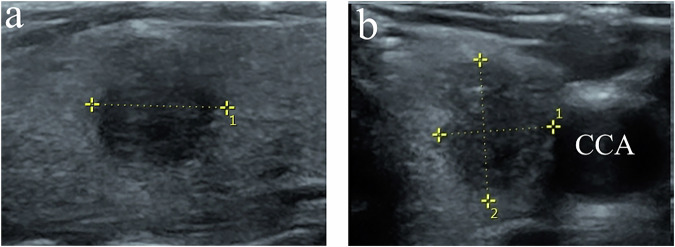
The longitudinal (a) and transverse (b) planes of 2D-US showed a 0.9 cm × 0.7 cm × 0.9 cm solid thyroid nodule in the left lobe of the thyroid. 2D-US, two-dimensional ultrasound; CCA, common carotid artery
